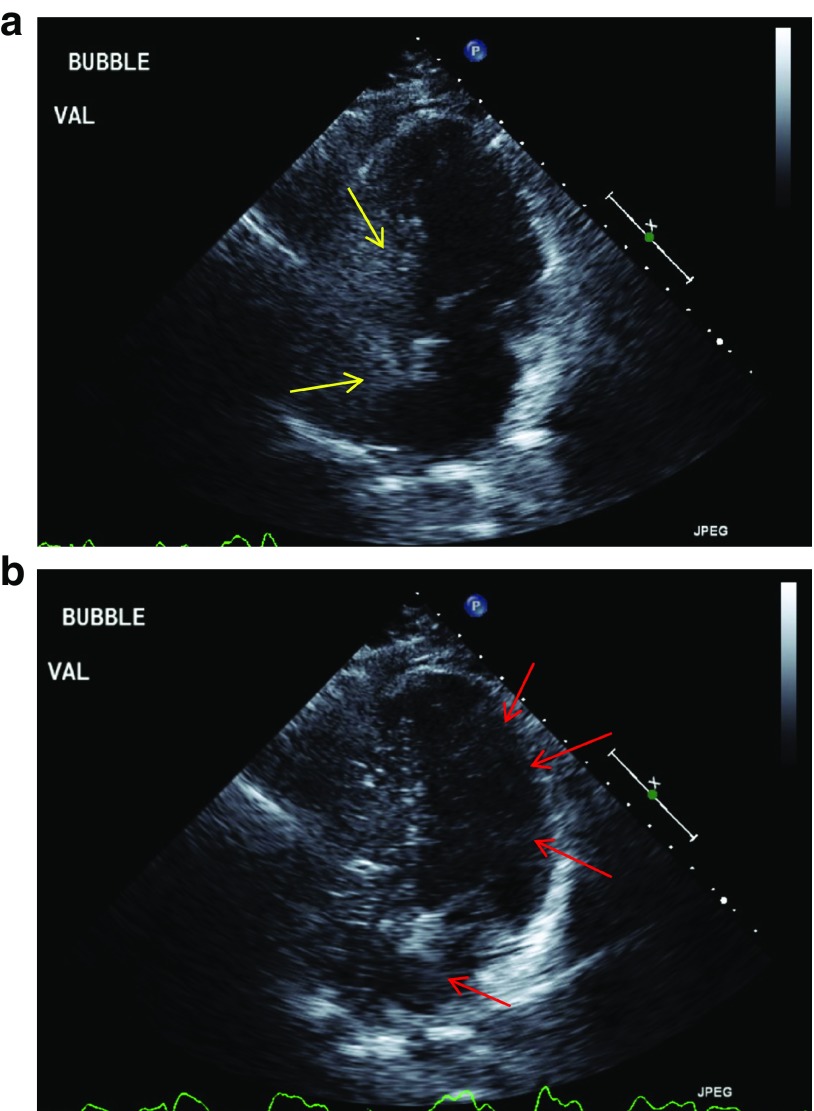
Still images from patient’s transthoracic echocardiogram showing (
